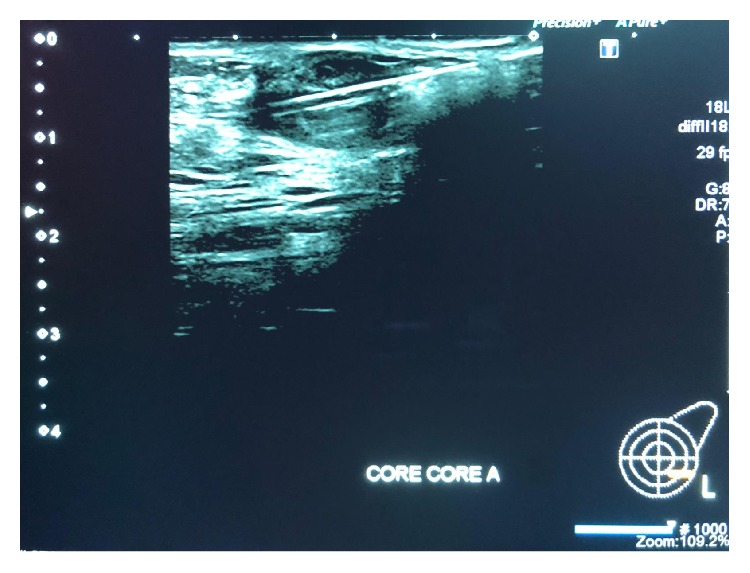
Ultrasound-guided core biopsy image of the first lesion (14-gauge needle).
